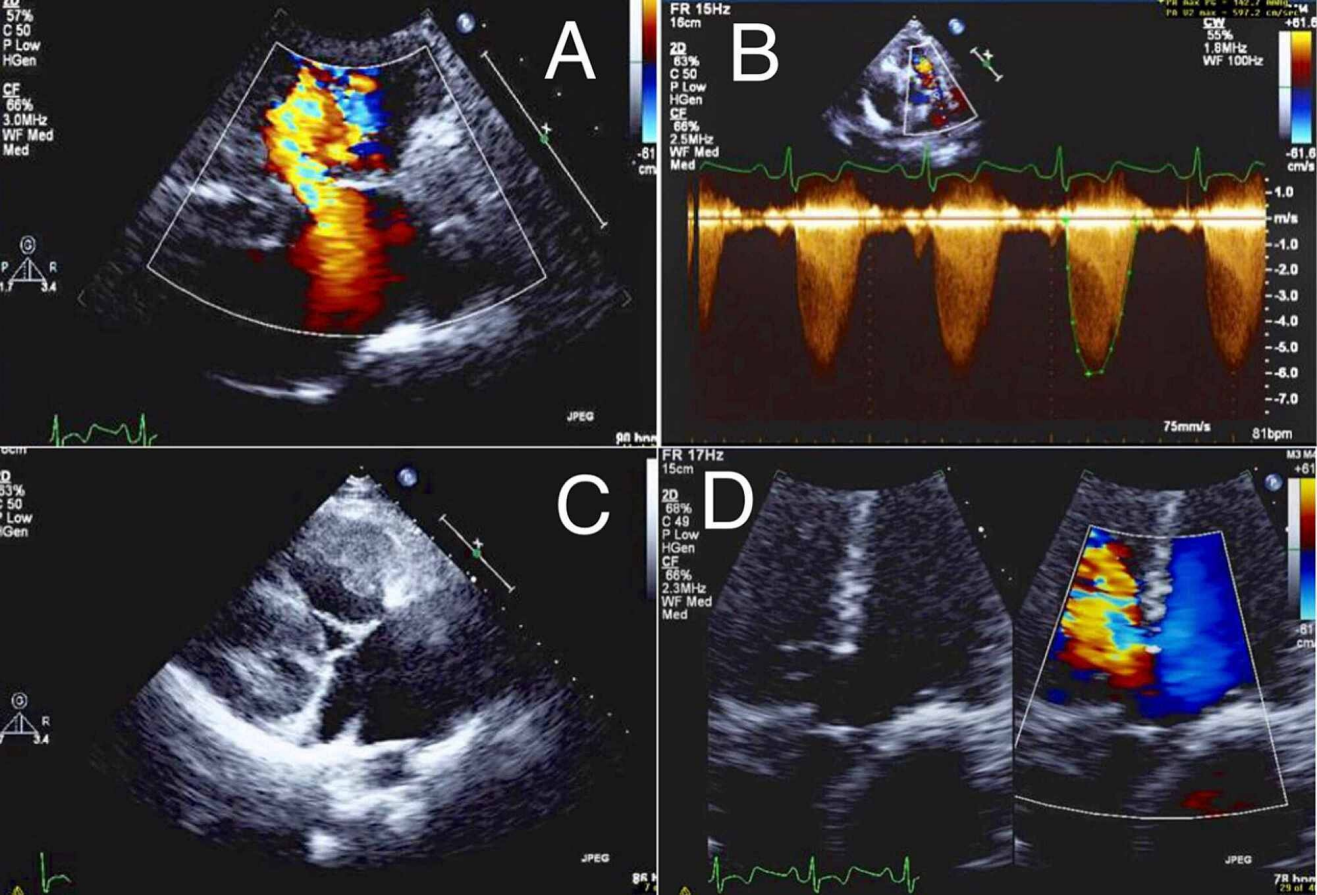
Patient’s echocardiogram showing (A) membranous ventricular septal defect with a right to left shunt, (B) severe pulmonic valve stenosis with a presence of dynamic obstruction, (C) severe right ventricular hypertrophy, and (D) overriding aorta.
